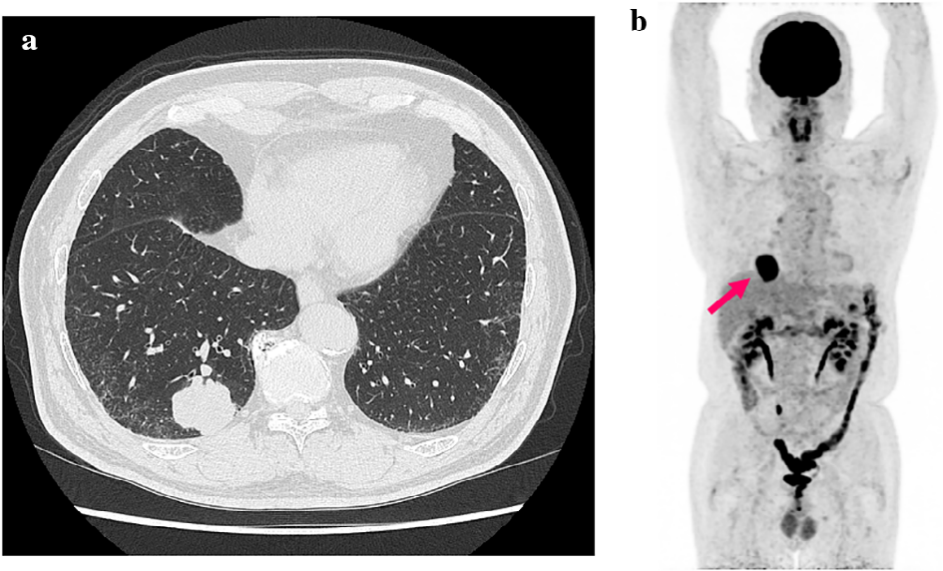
(a) Chest computed tomography (CT) scan revealed an abnormal mass in the right lower lobe. (b) ^18^F‐fluorodeoxyglucose positron emission tomography (FDG‐PET) shows a high uptake in this lesion.
